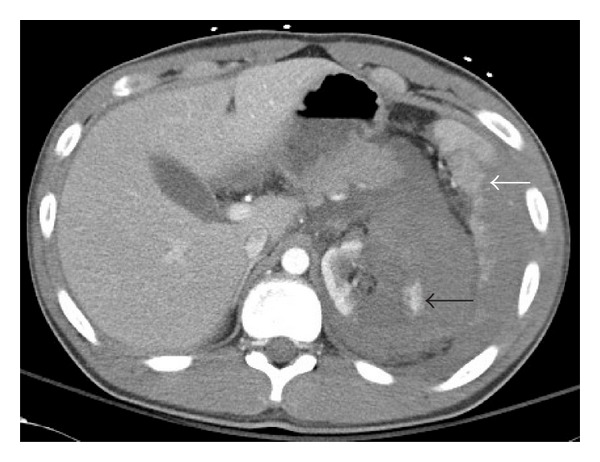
Axial CT of the abdomen revealing extensive left renal hematoma and laceration (white arrow). Also note the splenic laceration with subcapsular hematoma (black arrow).
